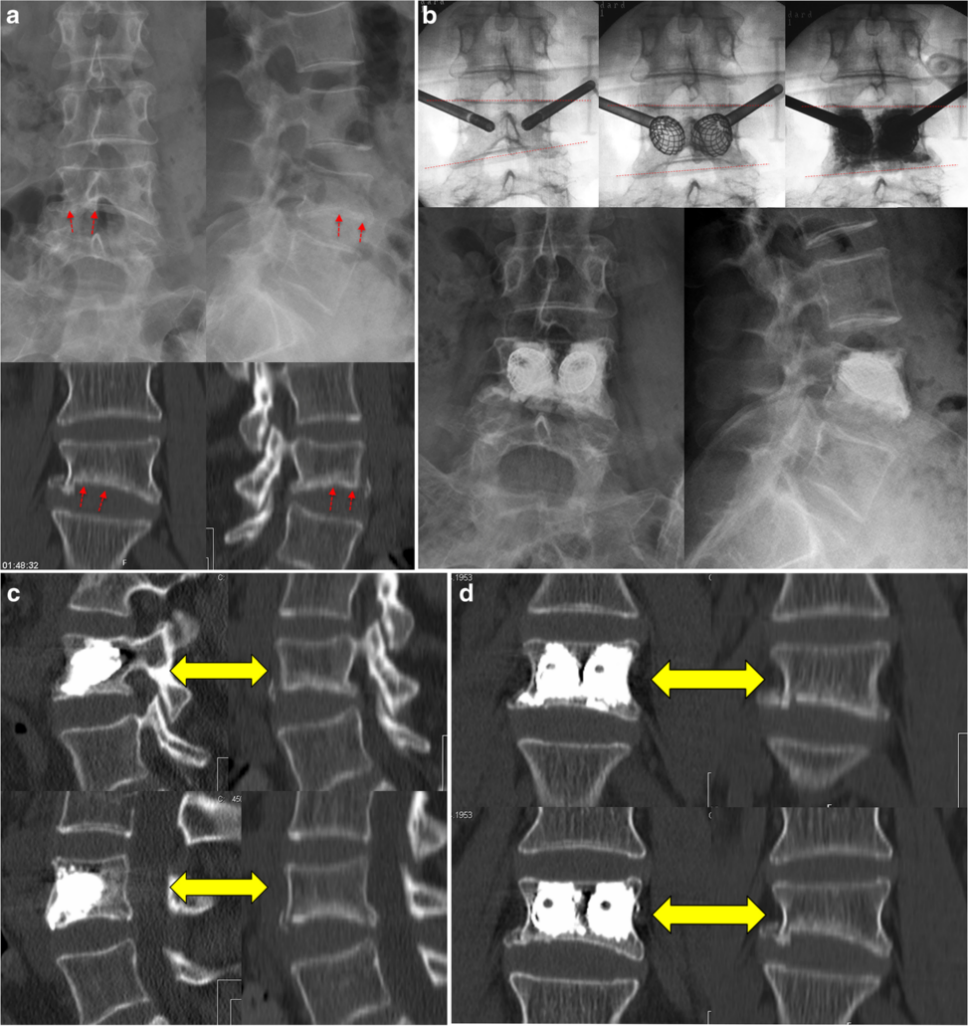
Case presentation. This 63 years old female presents after a minor car accident. Clinically the patient presents with right sided leg pain as soon as she gets up, however motor function is ok. The imaging studies depict an atypical fracture of L4 (Figure 2a), with an impression of the lower endplate. Obviously there is a foraminal stenosis at L4-L5 which provokes the leg pain. Because of the neurologic symptoms a surgical treatment was advocated. Instead of an open intervention a stentoplasty procedure was performed. The correction achieved is well visible by the intra- and postoperative pictures (dashed lines, Figure 2b). Compared to preoperatively the postoperative CT scan confirms an important correction that could be achieved by the stent and the well performed cement augmentation that stabilizes the vertebral body (Figures 2c,d).
